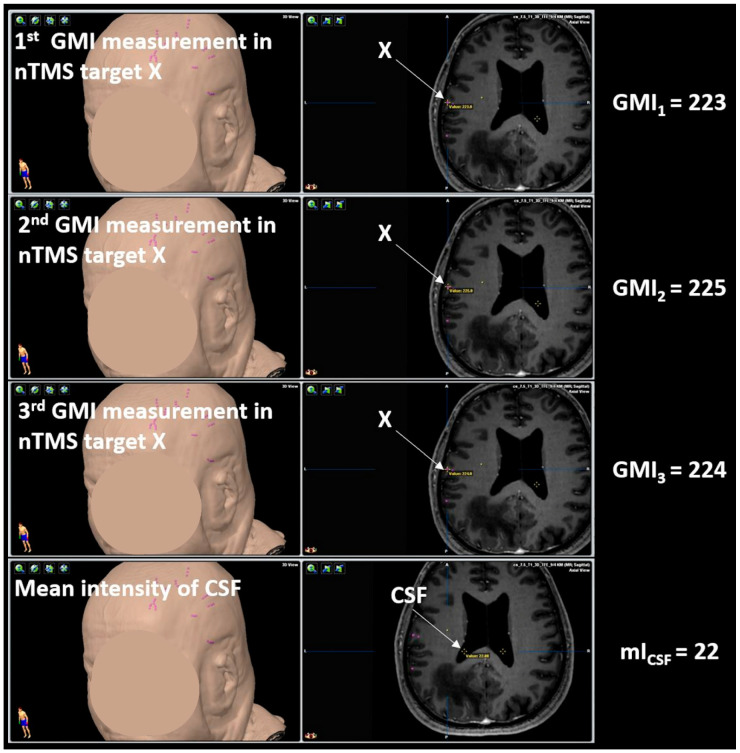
Analysis of gray matter intensity (GMI). The GMI of language-positive or language-negative spots, determined by language mapping using navigated transcranial magnetic stimulation (nTMS), was measured in T1-weighted sequences. Three pixels were randomly selected within a stimulation spot and used to calculate a mean GMI for language-positive nTMS spots (mGMI_p_) and language-negative nTMS spots (mGMI_n_). The signal intensity ratio (IR) was then calculated by dividing the mGMI_p_ or mGMI_n_ by the mean intensity of cerebrospinal fluid (mI_csf_).
